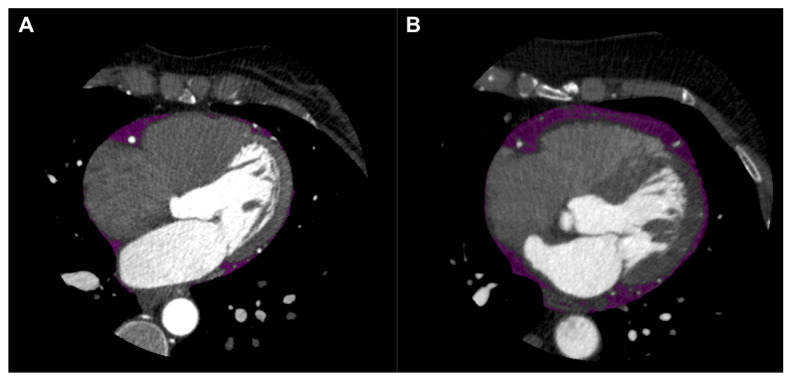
Epicardial adipose tissue (EAT) shown in purple on axial view of coronary computed tomography angiography (CTA). EAT in patient without coronary artery disease (CAD) shown in left panel (A), and EAT in patient with CAD shown in right panel (B).
